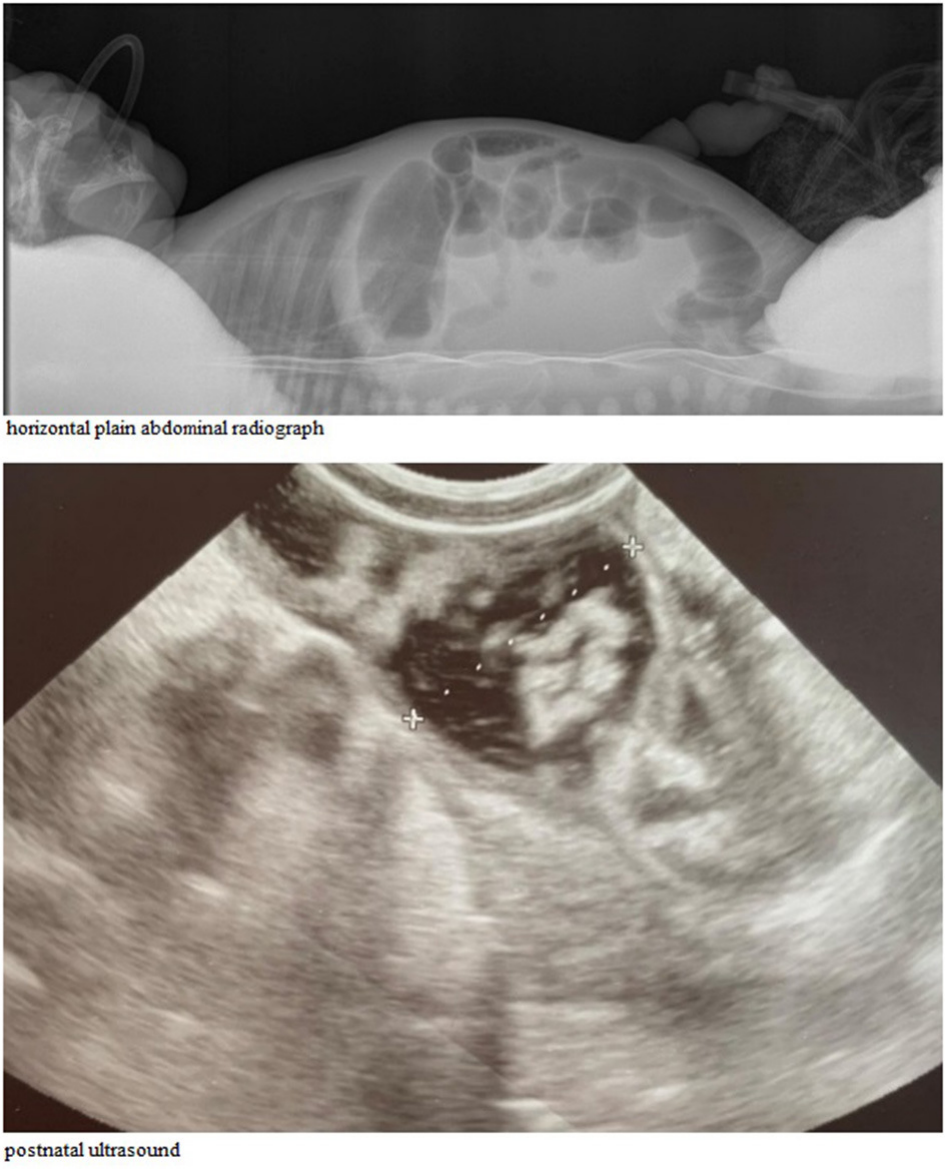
Abdomen X-ray in the first day of life, horizontal radius (Top). Abdominal ultrasound examination showing significantly distended intestinal loops of the newborn (Lower).
